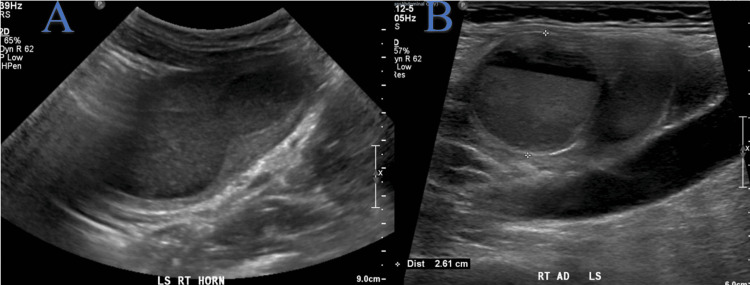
Longitudinal view of transabdominal ultrasound showing (A) right uterine horn with right hematometra and (B) right hematosalpinx
